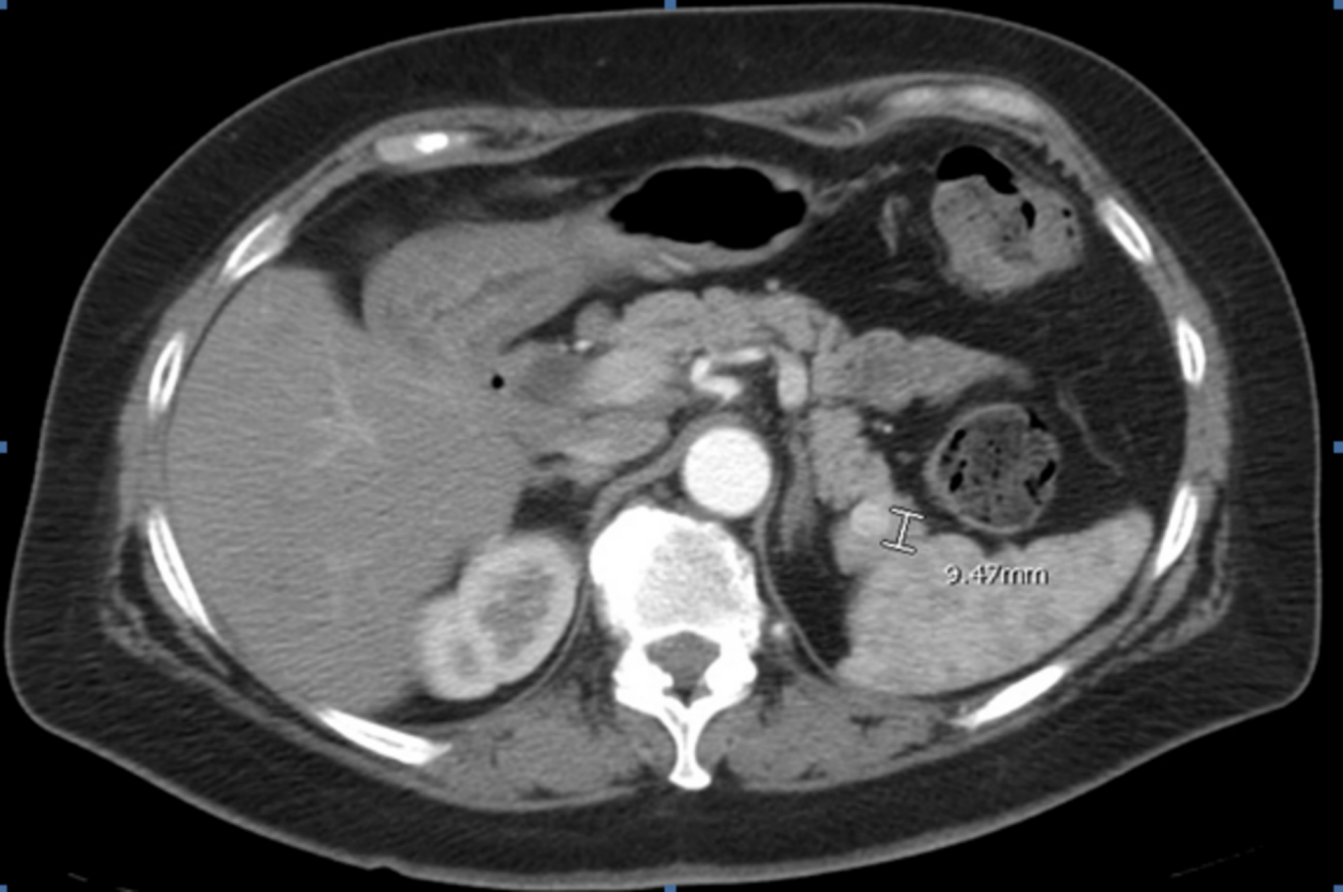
Year-nine post-nephrectomy imaging showed a single pancreatic tail lesion. CT of the abdomen and pelvis demonstrated a hypervascular focus demonstrated within the distal aspect of the pancreas measuring 0.9 cm. At that time, the enhancing focus within the tail of the pancreas appeared of the same density as the mesenteric arteries on that particular examination and was suspected to reflect a small splenic artery aneurysm.
